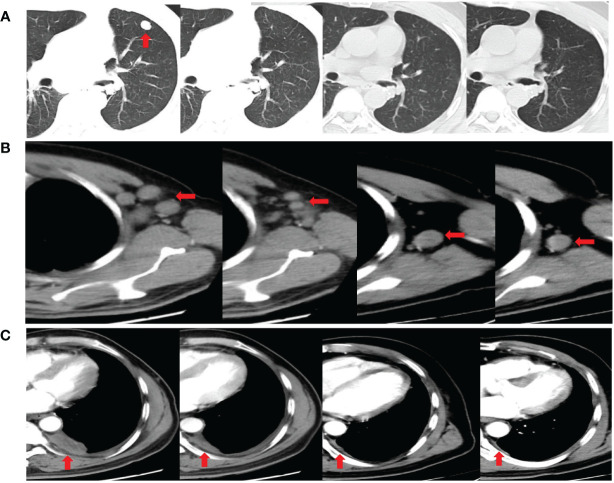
Chest computed tomography images showing the disappearance of the left lung metastasis (A), the shrinkage of the left axillary lymph nodes (B), and the shrinkage of the left pleural dissemination (C) after completing six cycles of tislelizumab plus etoposide and cisplatin induction therapy. Chest computed tomography images demonstrating a durable response after completing ten doses and fourteen doses of tislelizumab maintenance phase (Partial axillary lymph nodes disappeared after ten doses of tislelizumab).
